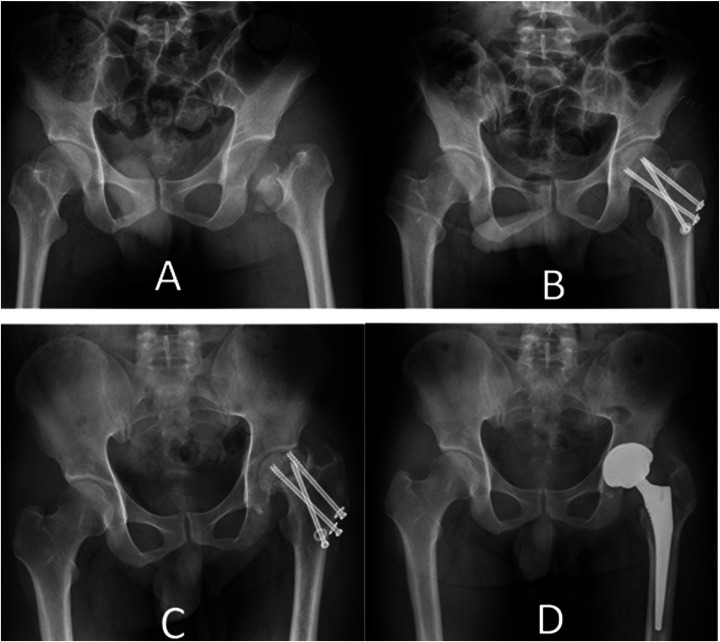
40-years old man sustained a high-speed vehicle accident (A) the pre-operative x-ray films revealed a pipkin III fracture involving the femoral head and neck on the left side. (B) The post-operative radiograph displayed internal fixation using three-cannulated screws. (C)Follow-up radiographs taken 18 months later indicated obvious implant failure and femoral head necrosis. (D) A postoperative radiograph taken two months after total hip arthroplasty is shown.
